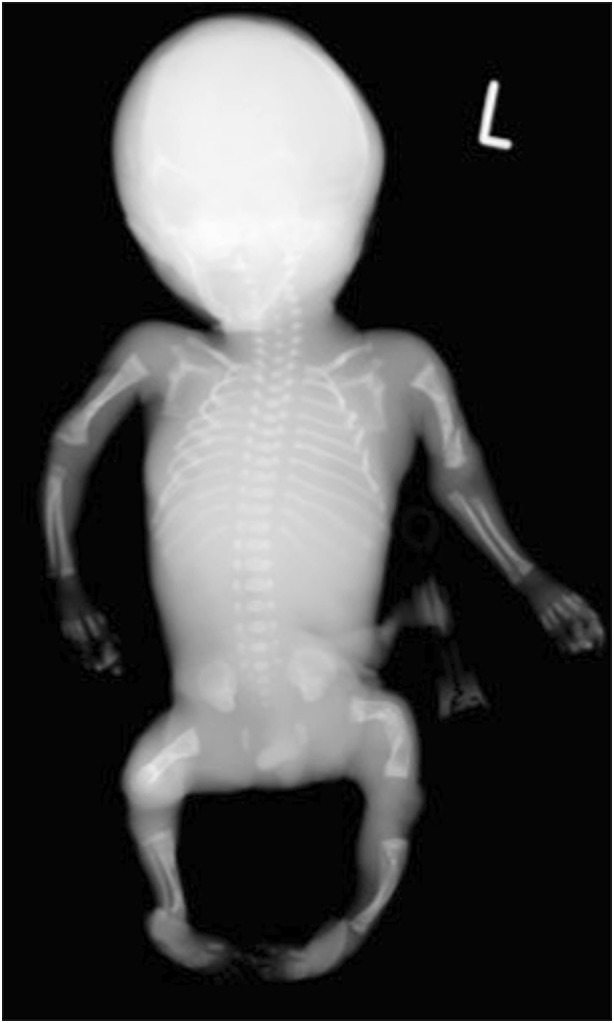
Radiographic features in a 22 weeks’ gestation male fetus with lethal osteogenesis imperfecta type II*.* Anteroposterior babygram shows generalized osteopenia, deformities of the ribs, and absent ossification of the skull vault
